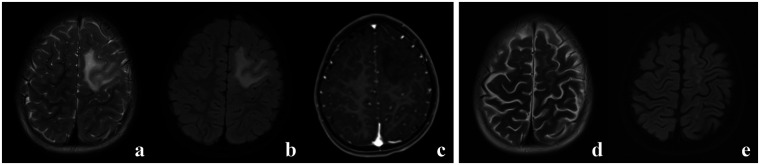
Brain MRI. Hyperintensity signal could been seen on T2-weighted (a) and FLAIR (b) in the left frontal region without enhancement on T1, plus gadolinium (c). Follow-up MRI performed 3 months after EPC onset showed a significant decrease in the area of hyperintensity on T2-weighted (d) and FLAIR (e).
